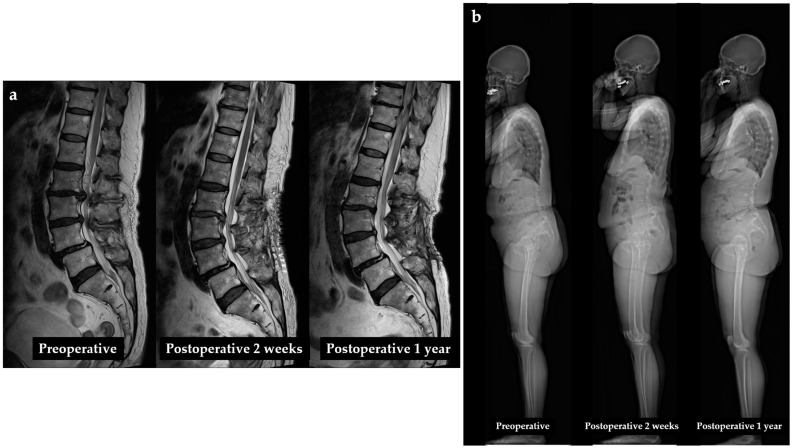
An 80-year-old female patient undergoing decompression for lumbar spinal stenosis: (a) magnetic resonance imaging shows lumbar spinal stenosis and decompression of the spinal canal after surgery; (b) EOS imaging shows preoperative sagittal imbalance and improvement of sagittal alignment at two weeks and one year postoperatively.
